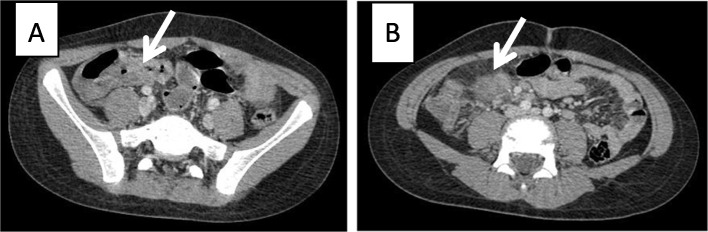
Axial contrast-enhanced CT images show circumferential wall thickening of the terminal ileum and ileocecal junction (thick arrow in A) and prominent inflammatory mesenteric lymph nodes with adjacent fat stranding (thick arrow in B)
